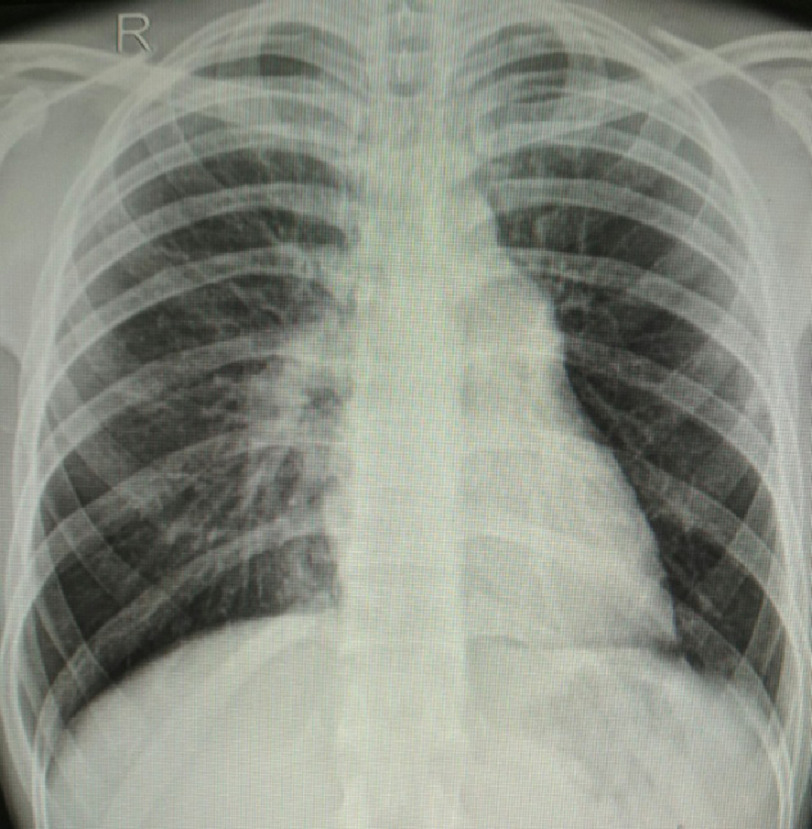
Chest radiograph showing a dilated main pulmonary artery and right pulmonary artery with reduced vascular markings in the left hemithorax.
